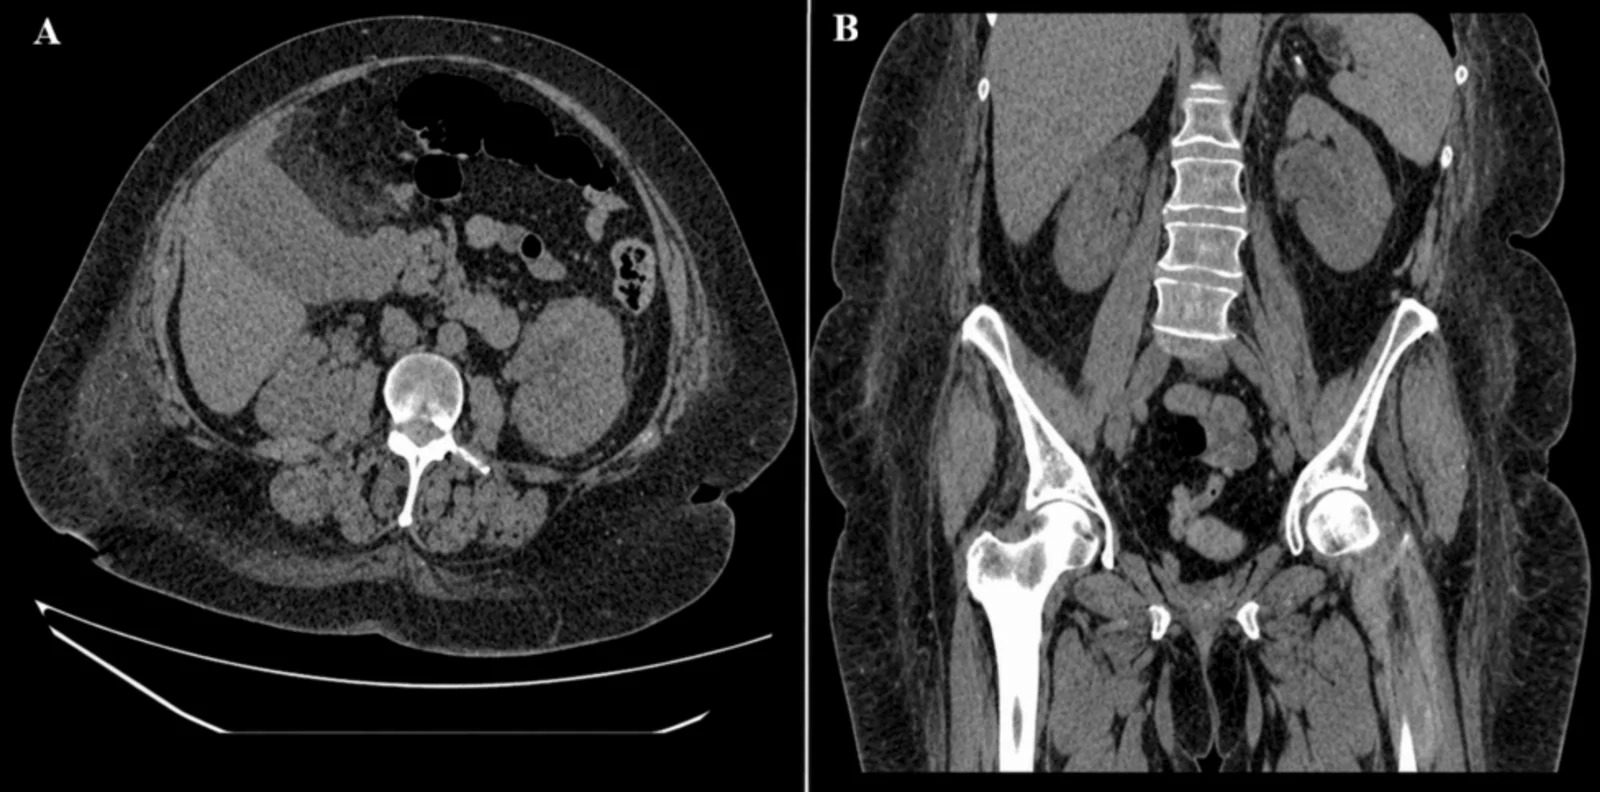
(A) Axial and (B) coronal views of CT abdomen The image shows the resolution of hydronephrosis post-catheter insertion CT: computed tomography
